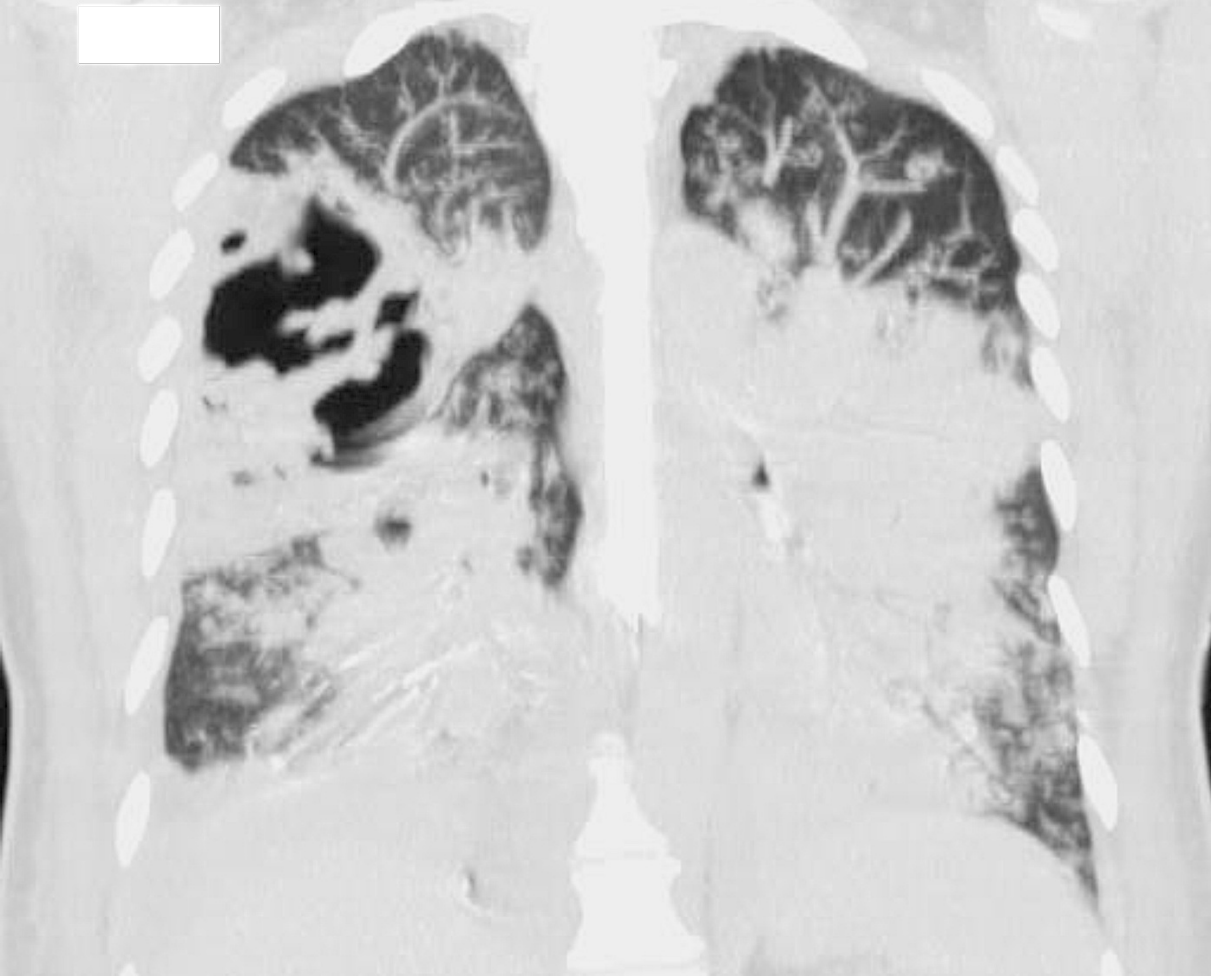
Repeat computed tomography (CT) chest (coronal view) showing worsening bilateral pneumonia.
